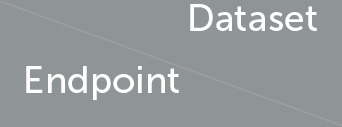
All (the whole dataset)	MRI	US	Other modalities
